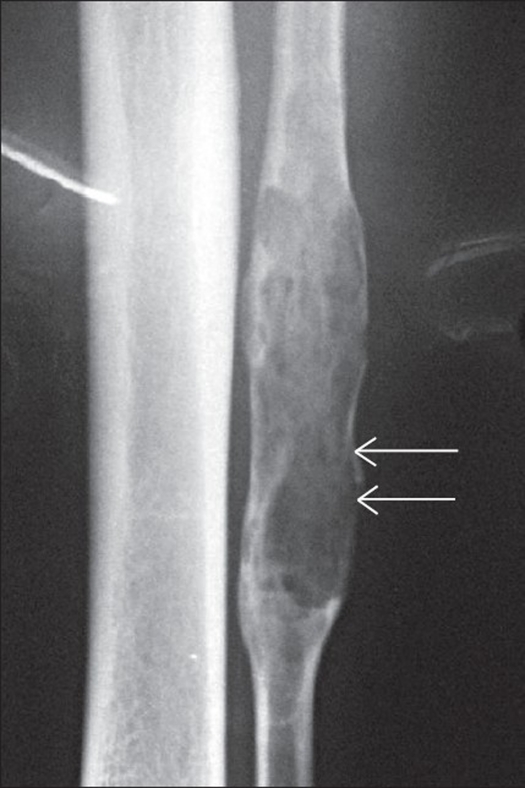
Plain radiograph (Antero Posterior view) of left fibula in a 42-year-old man showing a large cystic lesion with cortical thinning and no soft tissue extension
